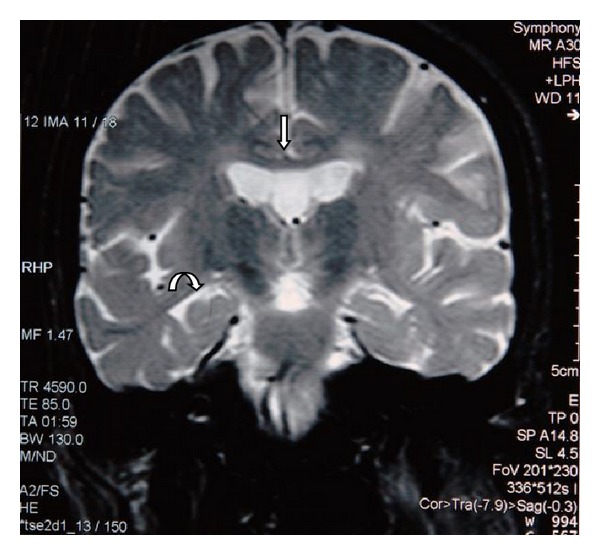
Coronal T2 weighted MR image of the brain shows bilateral hippocampal atrophy (curved arrow). Thinning of the corpus callosum also noted on this image with no evidence of signal intensity change (straight arrow).
